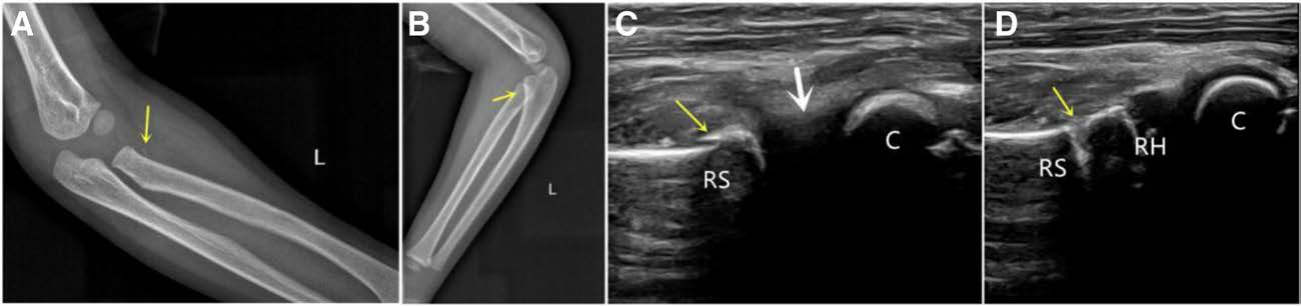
(A) A 5-yr-old boy, the displacement fragment was barely visible (yellow arrow) on the anteroposterior radiograph film. (B) There was an overlapping fracture shadow on the lateral film behind the proximal ulna (yellow arrow). (C) The sagittal longitudinal ultrasound scan showed obvious displacement of the radial head and a metaphyseal fracture line (yellow arrow), but the radial head was disappeared (white arrow). (D) Ultrasound showed normal “double-hump sign” and “congruency sign” after closed reduction. C = capitellum, RH = radial head, RS = radial shaft.
